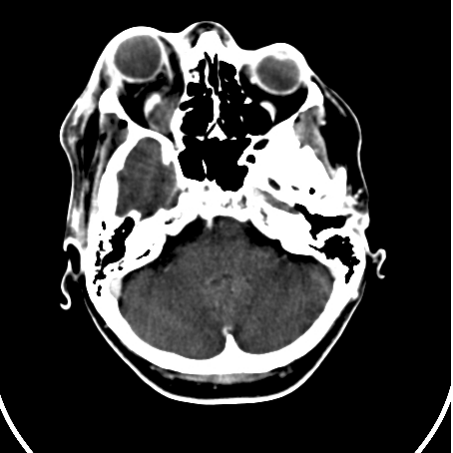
CT scan of the orbits and brain, axial view. Streaky increased density was noted within the intra-coronal and extra-coronal fat, and there was right proptosis.
